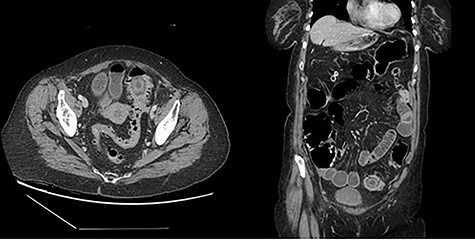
Representative cuts of Patient 1 CT scan.
